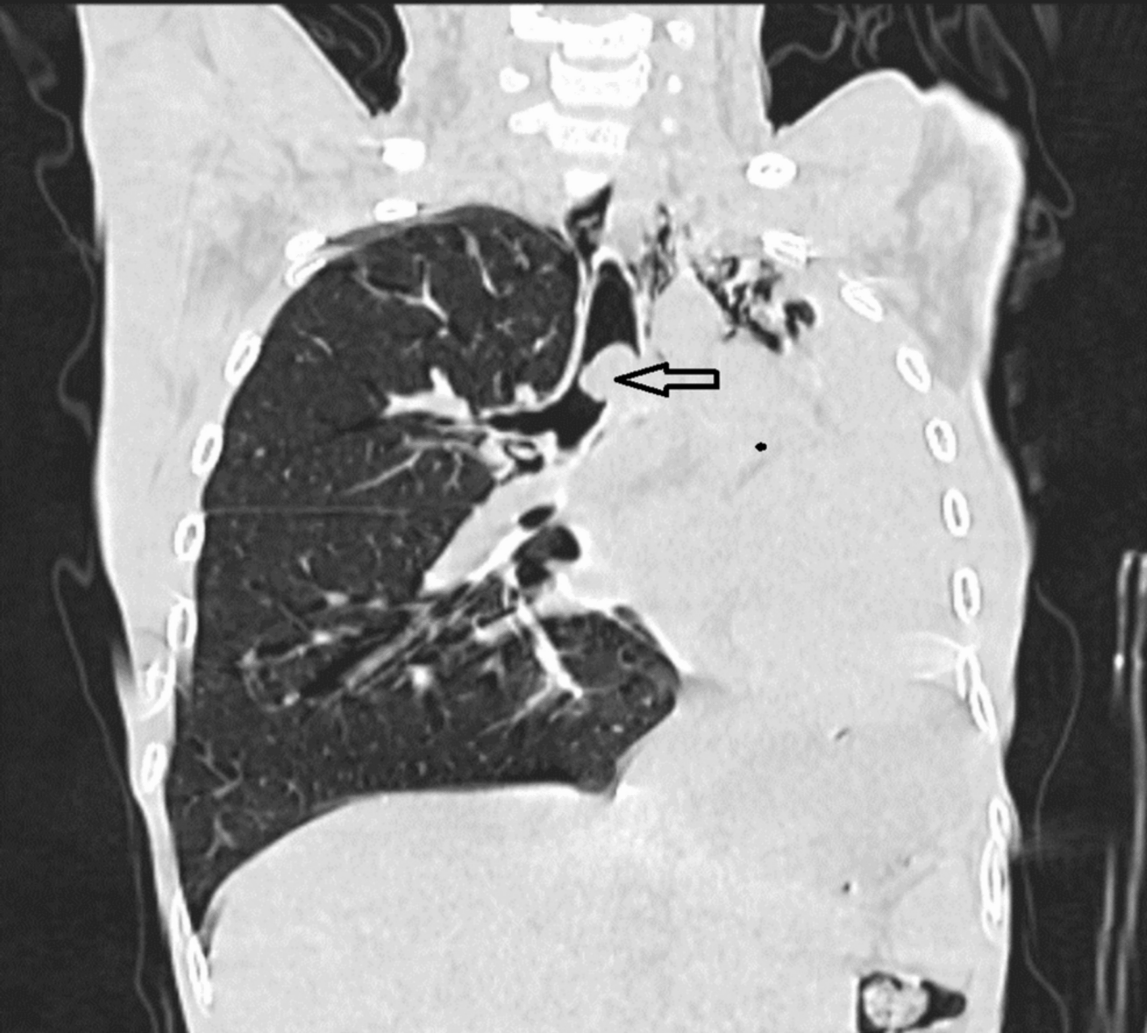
CECT of thorax showing a well-defined soft tissue density at the level of the tracheal bifurcation (black arrow) with lung collapse on the left side and compensatory hypertrophy on the right side CECT: contrast-enhanced computed tomography
